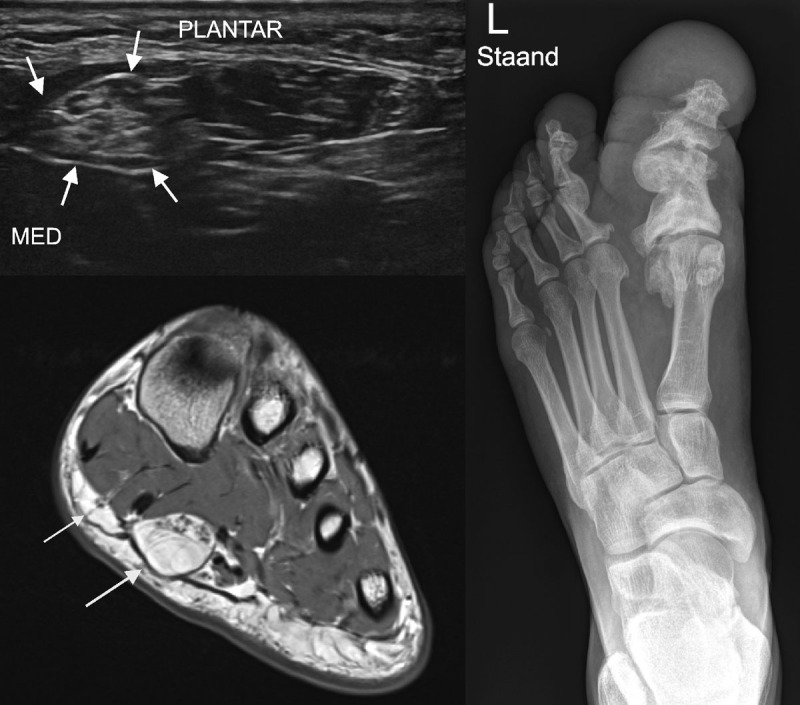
Left top image. US. Enlarged medial plantar nerve (arrows) in short axis next to flexor digitorum brevis in patient 2. Left bottom image. MR image in exactly the same location. The thickened medial plantar nerve can be seen as well as the medial hallucal nerve (arrow). Right image. Radiography. Macrodactyly in patient 2. The hallux is markedly enlarged with an increase in soft tissue and bony overgrowth in an irregular fashion.
